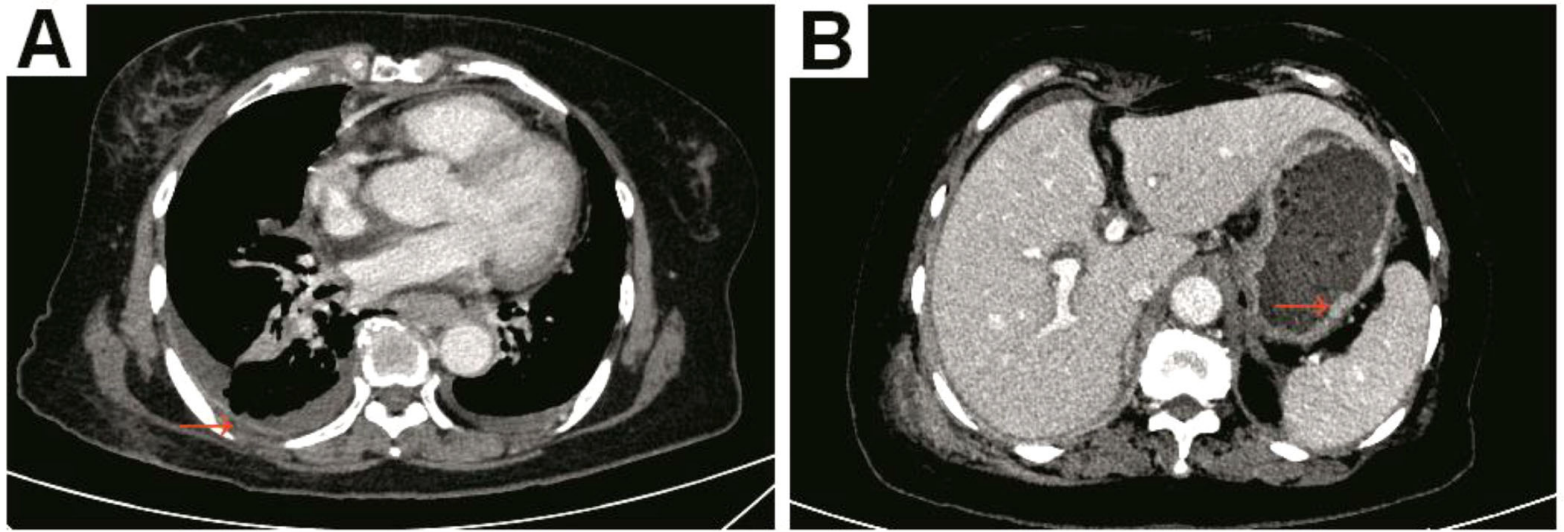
A chest enhanced computed tomography (CT) showed thickening of the right lateral parietal pleura (red arrow) with a small amount of pleural effusion (A); an abdominal enhanced CT revealed findings consistent with gastric wall thickening and indistinct layers of the dorsal wall of the gastric body, accompanied by marked enhancement of the gastric mucosa (red arrow) (B).
